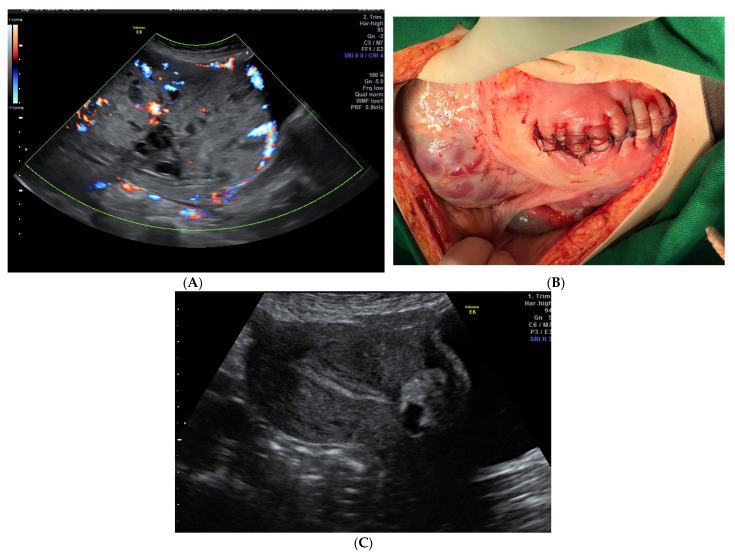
(A). Ultrasound appearance of the placenta at 35 weeks of pregnancy. (B). Placenta left in situ and corporeal-fundal uterine suture at delivery. (C). Transabdominal ultrasound examination 27 weeks after delivery. On the anterior uterine wall, the small placenta is noted, and the endometrium is linear. The patient had a history of CS and, at 20 weeks, she was diagnosed with placenta previa with a high suspicion of anterior abnormal invasion to the urinary bladder. She had no vaginal bleeding. Planned Caesarean section was performed at 36 weeks of gestation, with a vertical mid-line incision chosen for the abdomen and a fundal incision of the uterus to avoid the upper pole of the placenta. After the delivery of the baby, the decision to leave the placenta in situ was taken to avoid significant bleeding and bladder injury. After the ligature of the umbilical cord close to its placental insertion, the uterine wall was sutured (B). Close monitoring was offered and, at 27 weeks after delivery, the placenta was almost fully evacuated (C) and the patient had normal menstruation.
